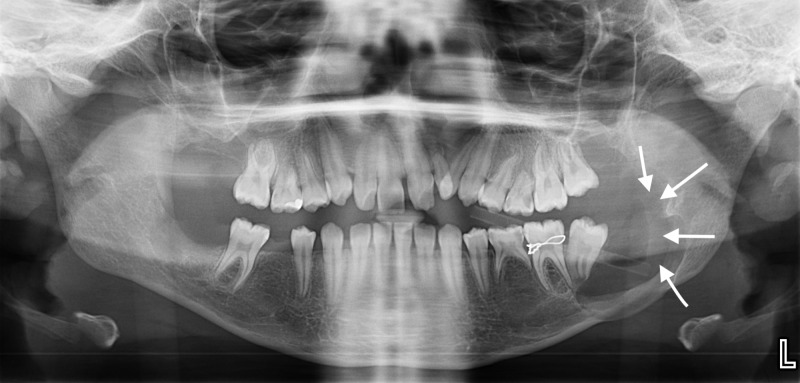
Orthopantomogram (OPG) after two months of follow-up. An orthopantomogram (OPG) after two months of follow-up shows improvement and bone deposition (white arrows).
